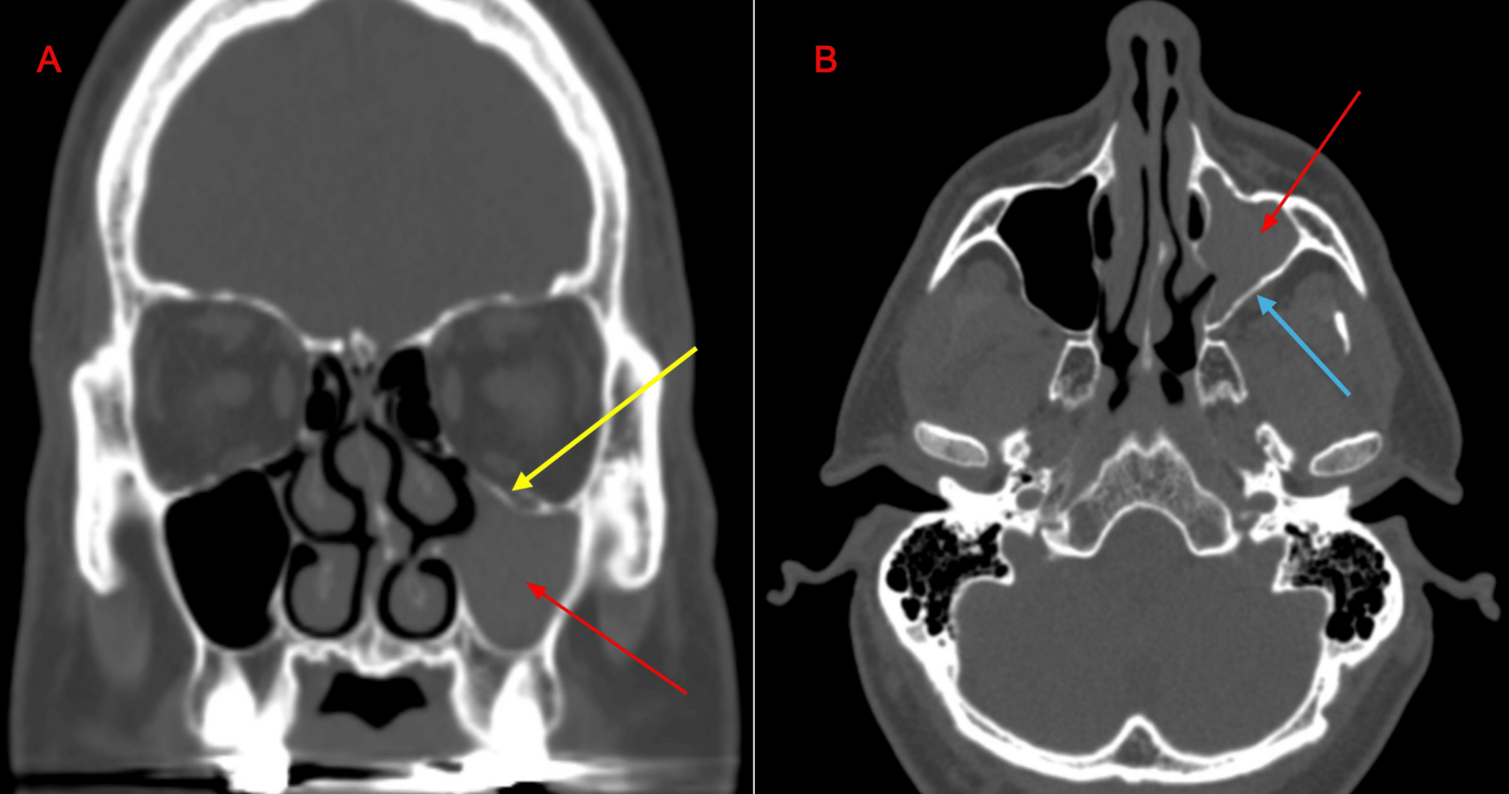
Non-contrast CT imaging of the paranasal sinuses showing left maxillary sinus opacification and associated structural changes. Coronal (A) and axial (B) views of a non-contrast CT scan of the paranasal sinuses demonstrating complete opacification of the left maxillary sinus (red arrows). Additional findings include atelectasis of the left uncinate process, bowing of the orbital floor (yellow arrows), and inward bowing of the posterior wall of the maxillary sinus (light blue arrow).
